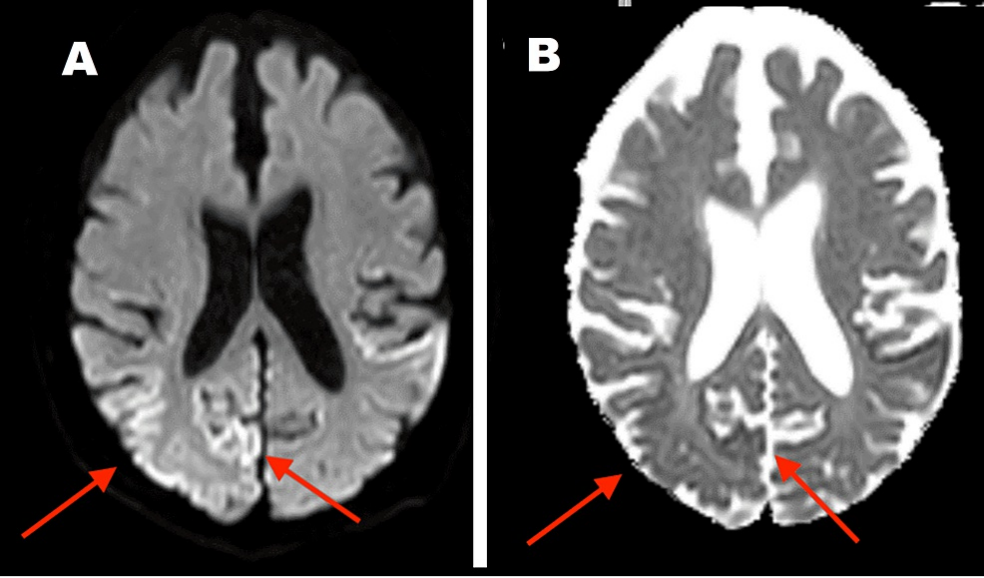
Initial MRI Brain shows cortical changes in the right occipital region (A) Diffusion-weighted imaging (DWI) sequence; (B) Apparent diffusion coefficient (ADC) sequence
